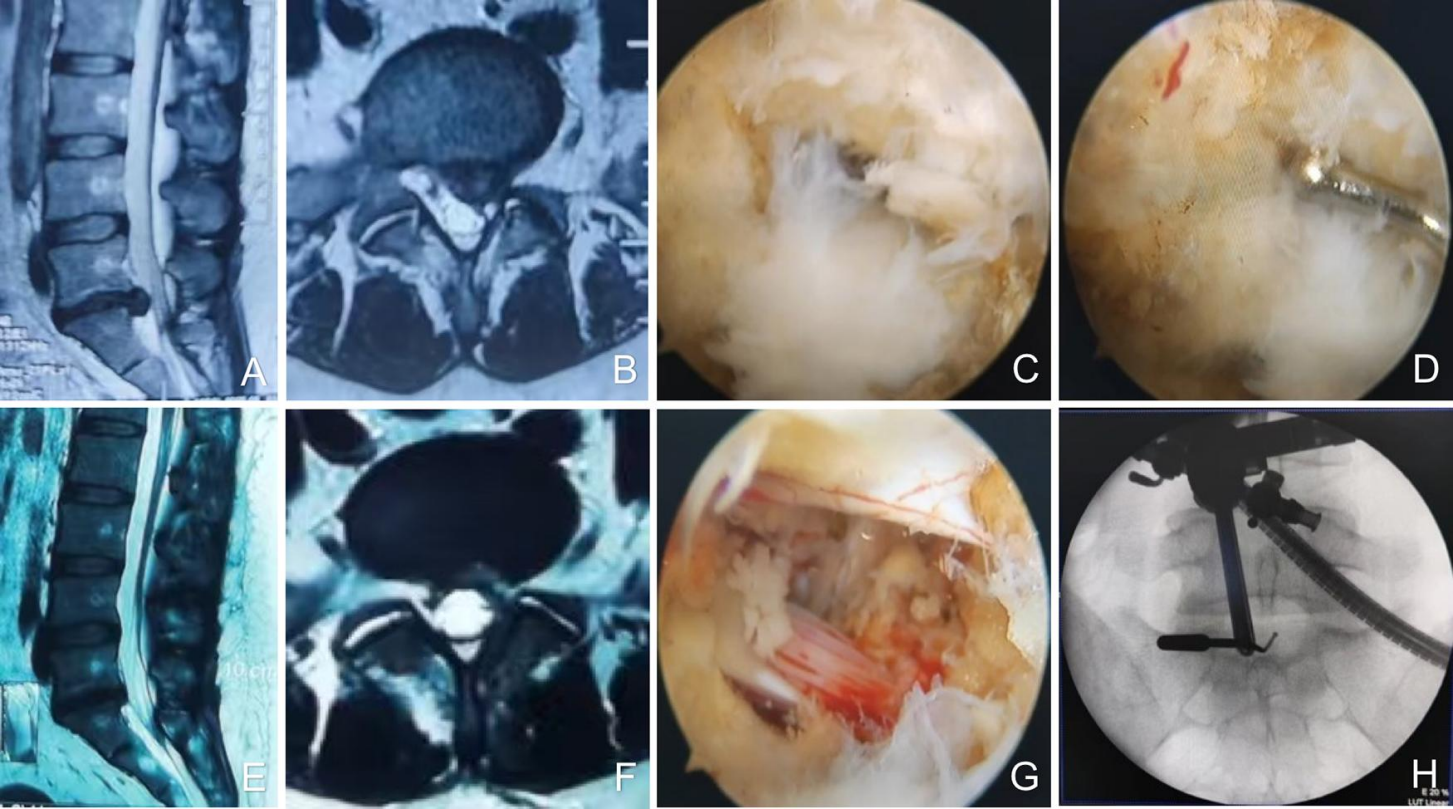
Female, 36 years old, L5/S1 lumbar disc herniation. (A) Preoperative sagittal MR image showed L5/S1 lumbar disc herniation; (B) preoperative axial MR image showed herniated lumbar disc compressed nerve root and dural sac; (C) intraoperative ligamentum flavum splitting line; (D) the fissure in the ligamentum flavum is only 2 mm; (E) postoperative sagittal MR image revealed the complete decompression of the spinal canal; (F) postoperative axial MR image showed the complete removal of herniated disc and bony fragment; (G) intraoperative image after complete neural decompression; (H) intraoperative fluoroscopy shows decompression crossing the midline.
